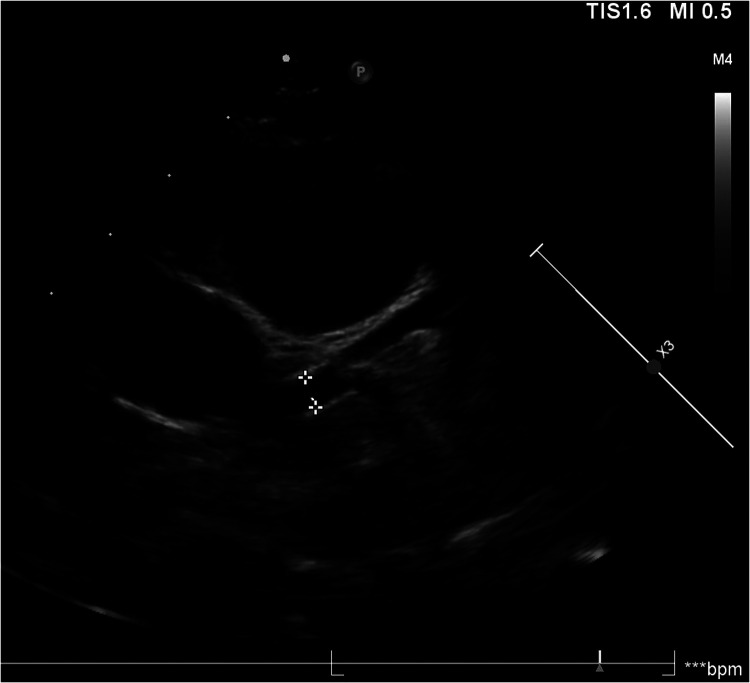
Images of small aneurysms in patient with concomitant kawasaki disease. On the 20th day of the disease course, the patient presented with fever, generalized diffuse rash, red and chapped lips, bayberry tongue, bilateral cervical lymph node enlargement and swelling of hands and feet, and diagnosed with KD. On the second day after the diagnosis of KD, echocardiography revealed that the left coronary artery small aneurysms (*Z* = 2.53).
